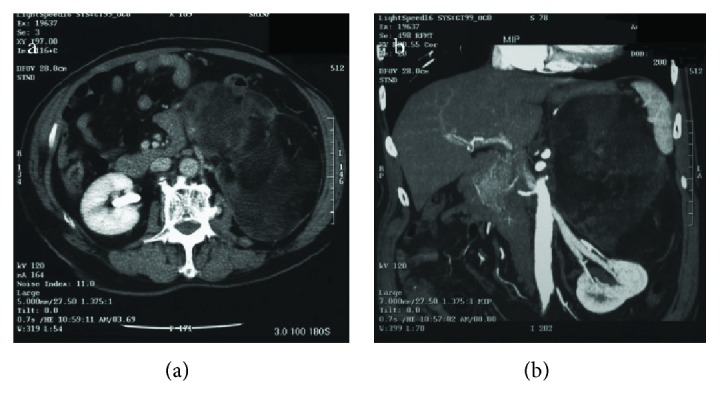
Abdominal computed tomography (CT) demonstrated a child-head-sized mass with heterogeneous contrast located in the left upper abdomen around the stomach, spleen, pancreas, and left kidney on the horizontal (a) and coronal (b) images.
